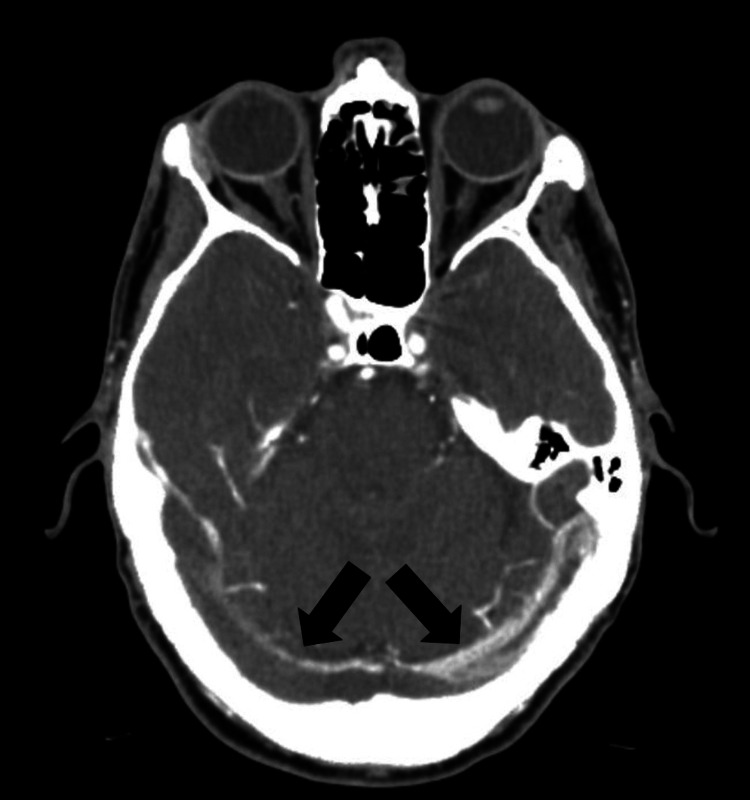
CT venogram demonstrating bilateral sigmoid sinus thrombosis (black arrows), with filling defects in both sigmoid sinuses, more pronounced on the right
